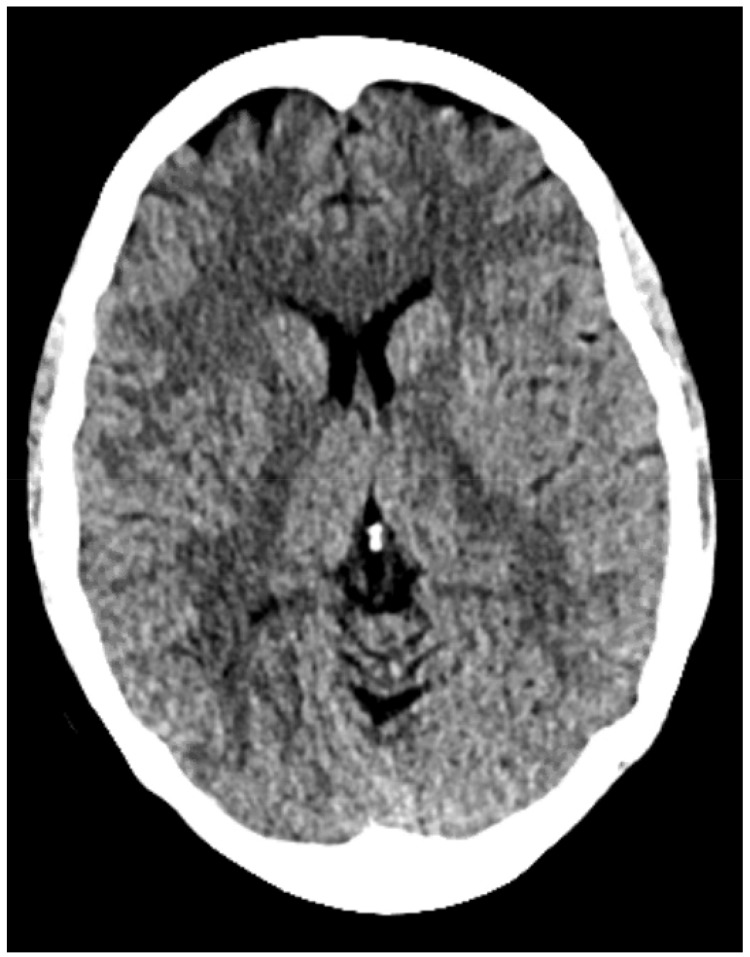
CT brain non-contrast performed after presentation for suspected CSF leak confirming no pneumocephalus.
